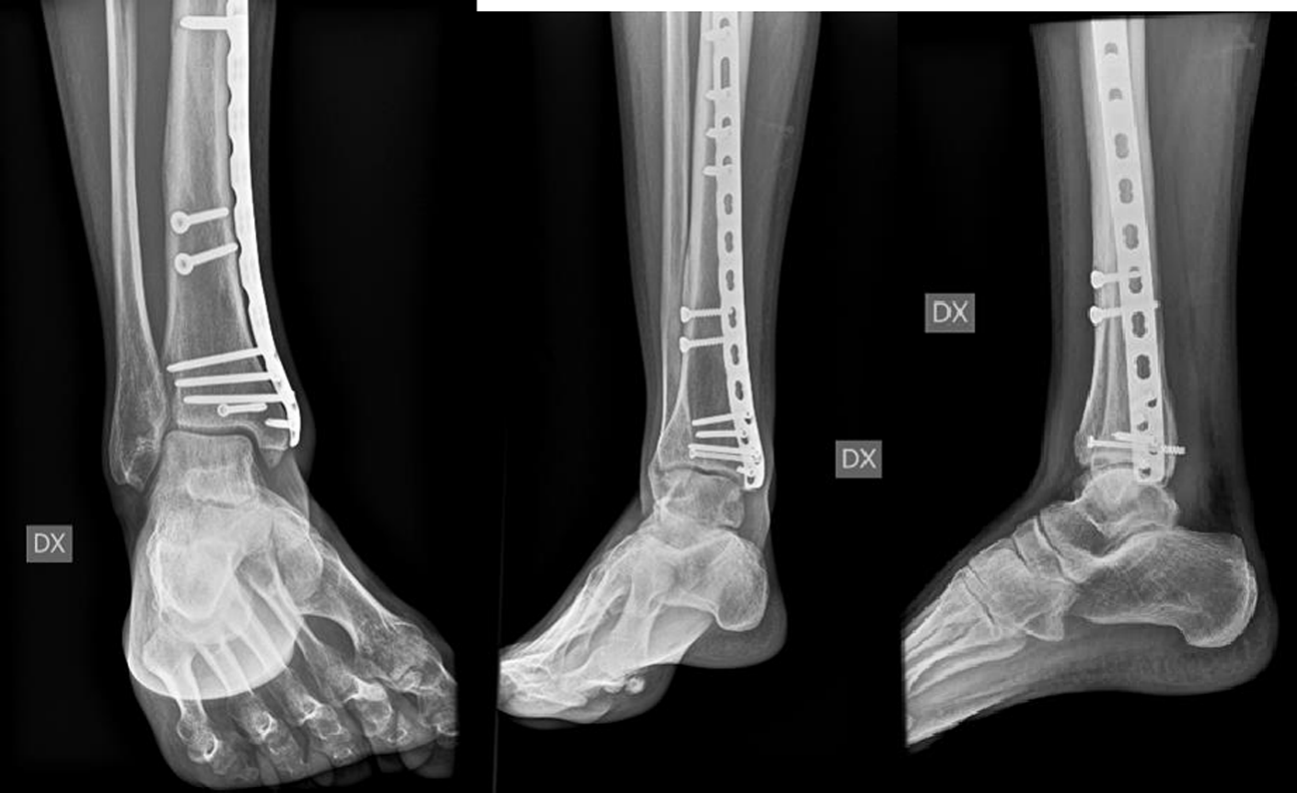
(a,b,c) Post-operative radiography at 6 months follow-up of LP patient with good formation of bone callus.
